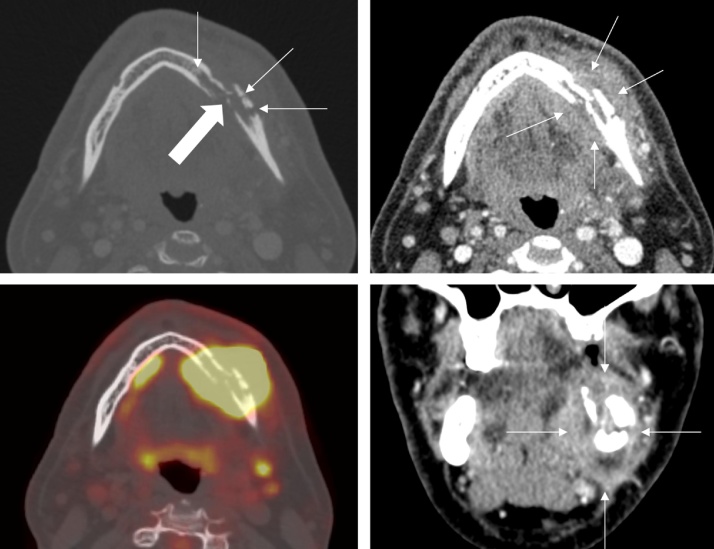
CT after i.v. contrast administration in transversal and coronal plane (upper row a, b; lower row right d) and fused PET-CT data (lower row left c), showing the fracture (thick arrow, a) as well as the underlying bone destruction of cortical and spongy bone (thin arrows, a) and the surrounding, slighty contrast enhancing soft tissue swelling (b) as well as the FDG-tracer uptake within the tumor and within the both ipsilateral suspected lymph node metastases.
